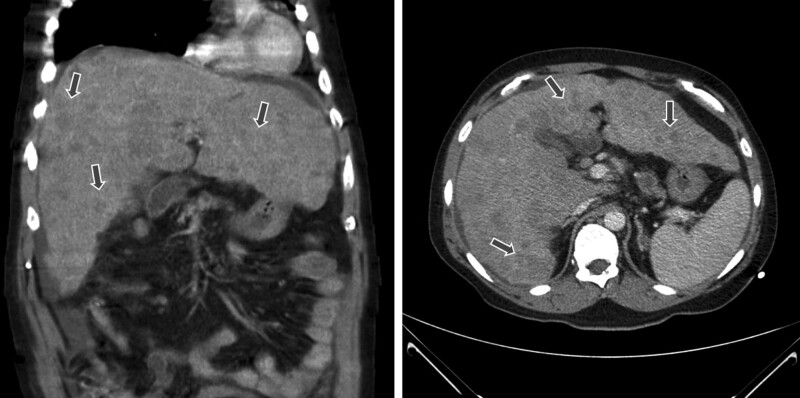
Chest computed tomography scan contains multiple foci (arrows) in both lobes of the liver.
